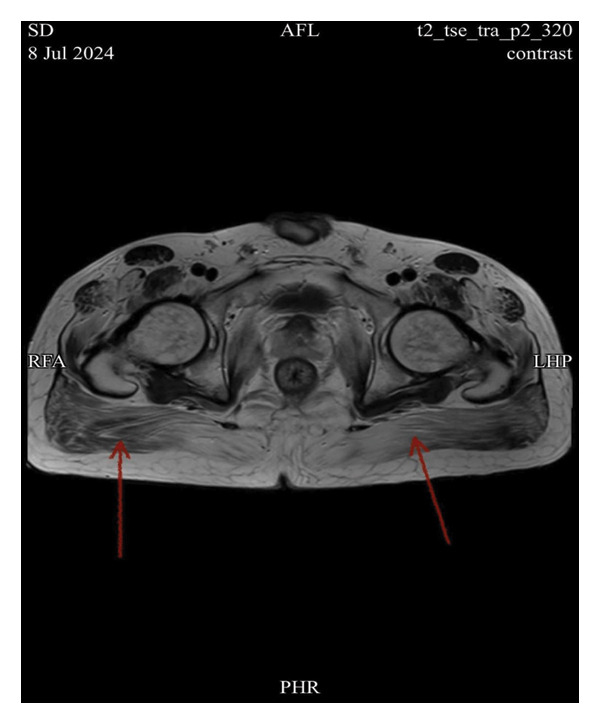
Axial T1‐weighted MRI image demonstrating diffuse atrophy and fatty replacement of the gluteal muscles (indicated by the red arrow).
